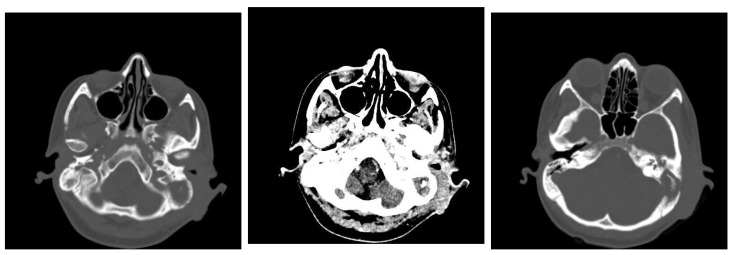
Patient with the diagnosis of chronic suppurative left exteriorized otomastoiditis. CT examination, in postcontrast CT axial sections, parenchymal window and bone window, with bone sequestration and fistulization in the subcutaneous soft parts.
